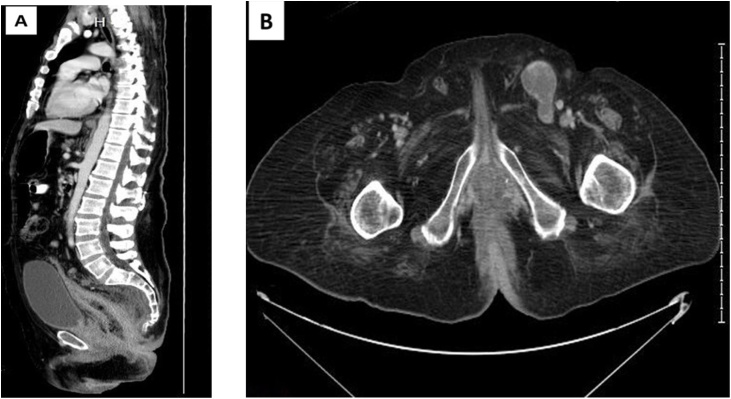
Sagittal and axial (B) reformatting for abdomen and pelvis CT scan show diffuse wall thickening of the colon with haziness of the meso-rectal fat planes and multiple regional enlarged lymph nodes. The thickening is extending into the anus. There are also multiple enlarged lymph nodes in inguinal areas.
